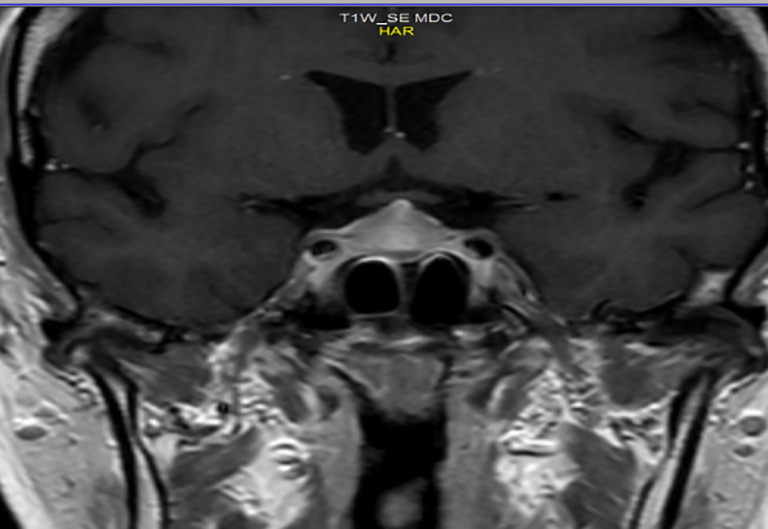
MRI: Radiological signs of hypophysitis. Inhomogeneous and enlarged appearance of the pituitary gland showing “tent” morphology due to tension of the meninges with thickening of the pituitary stalk.
